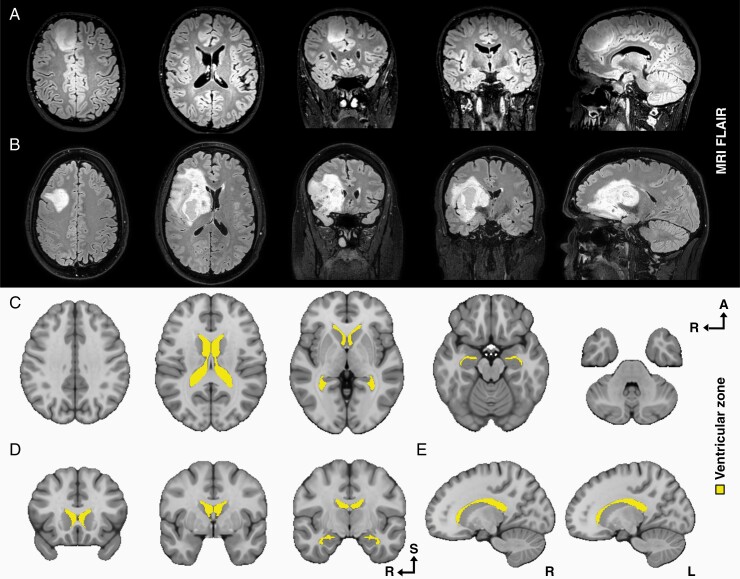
The images show examples of the qualitative assessment for the involvement of the subventricular zone in preoperative magnetic resonance imaging with fluid-attenuated inversion recovery and the anatomical definition of the ventricular zone used as target for contact and distance measurements in MNI space. (A) Axial, coronal, and sagittal slices of a tumor not in contact with the ventricular zone; (B) axial, coronal, and sagittal slices of a tumor in contact with the ventricular zone; (C) target for contact in axial slices (36, 18, 0, −18, −36); (D) target for contact in coronal slices (20, 0, −10); and (E) target for contact in sagittal slices (15(R) and −15(L)). MNI, Montreal Neurological Institute.
